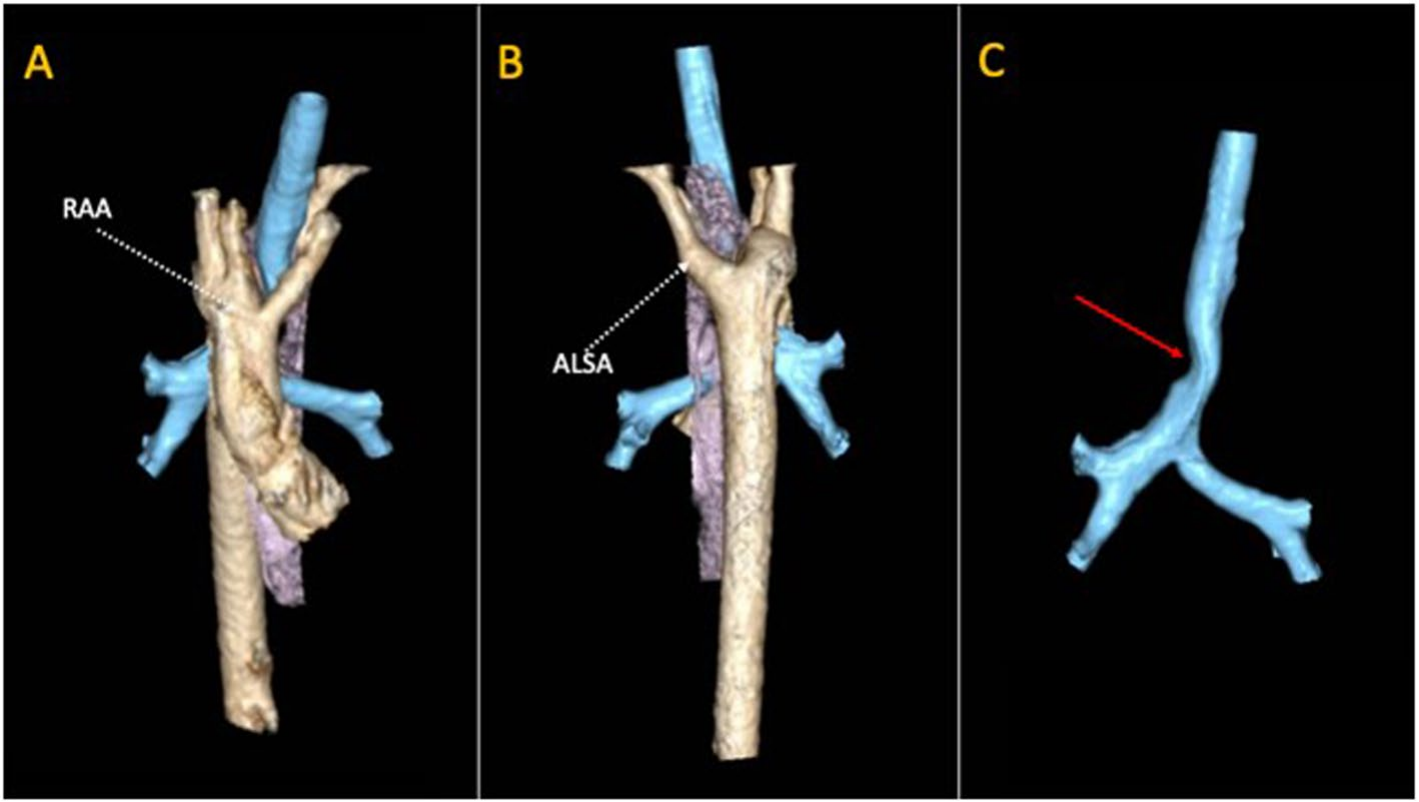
3D volume rendering reconstruction of a cardiac CT in patient with Right Aortic Arch (RAA) and Aberrant Left Subclavian Artery (ALSA). The vascular structures together with the left duct ligament (not visible on CT) completely encircle the trachea (in blue) and the oesophagus (in violet). Panel A: front view. Panel B: back view. Panel C: trachea with the narrowing (red arrow) at the level of the ring
